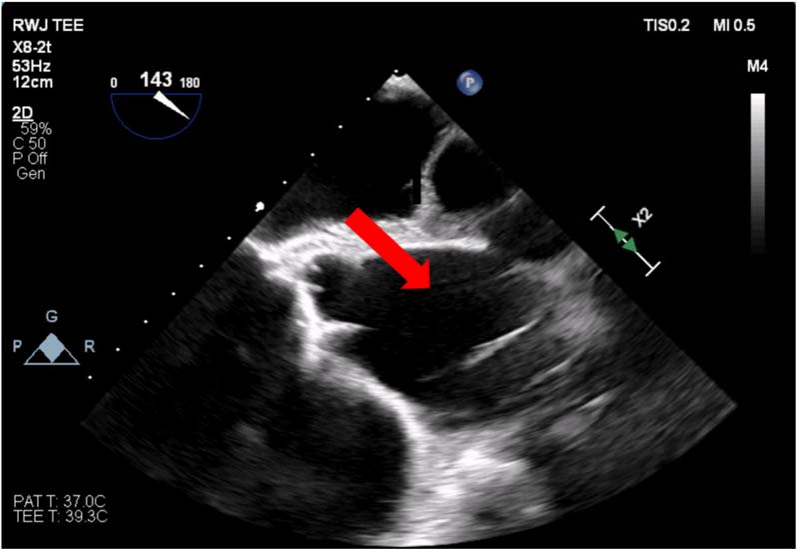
Transesophageal image of the aortic root and ascending aorta with IABP present.
